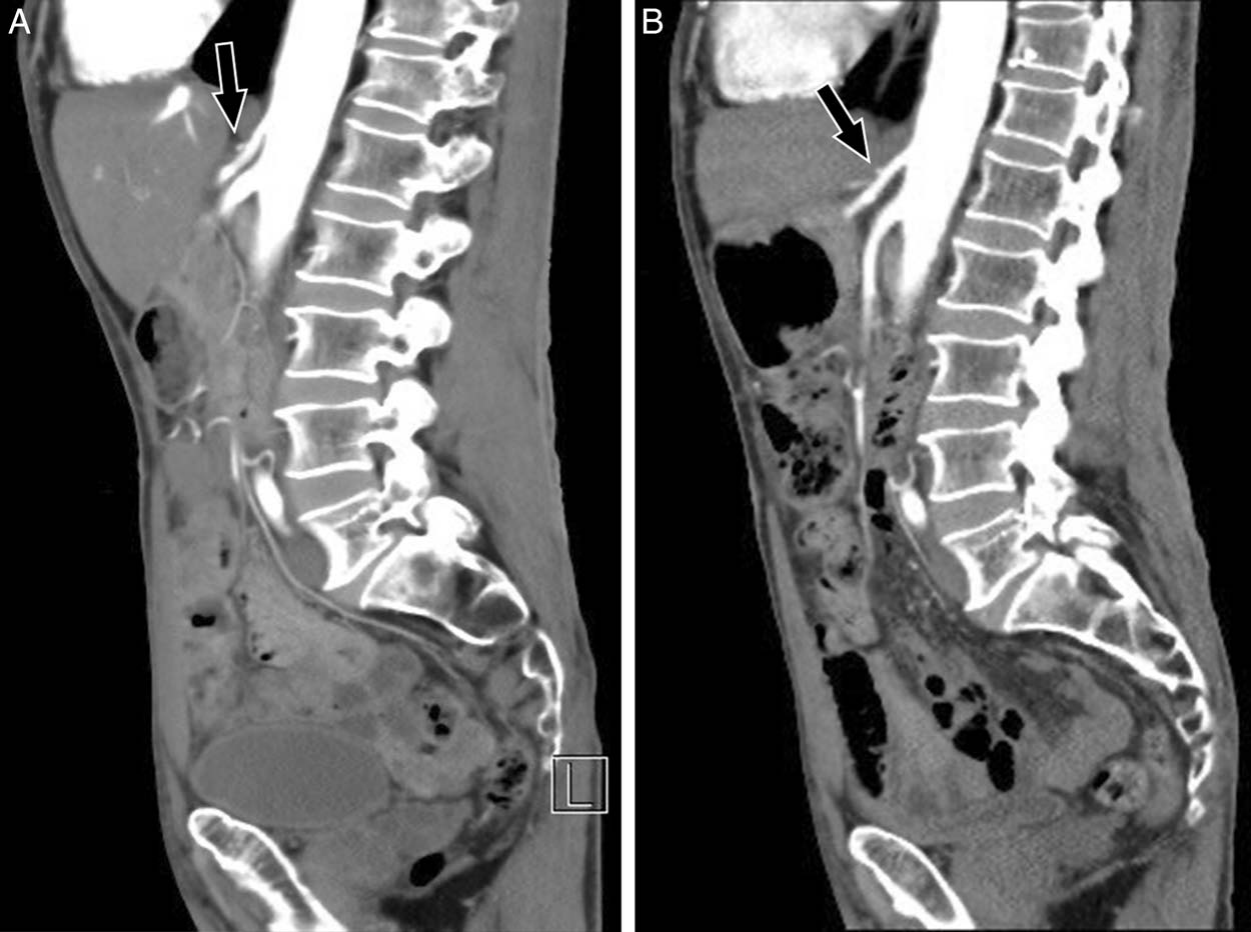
(A) Presurgical abdominal multi-slice computed tomography (MSCT) with contrast (sagittal view): focal mild compression of the celiac trunk is present. The celiac artery is slightly driven down (arrow) due to extrinsic compression by a thickened median arcuate ligament >7 mm (normal 4 mm). (B) Postsurgical abdominal MSCT with contrast (sagittal view): after laparoscopic release of the median arcuate ligament, the celiac trunk is shown in the normal position (arrow) with no observed stenosis.
